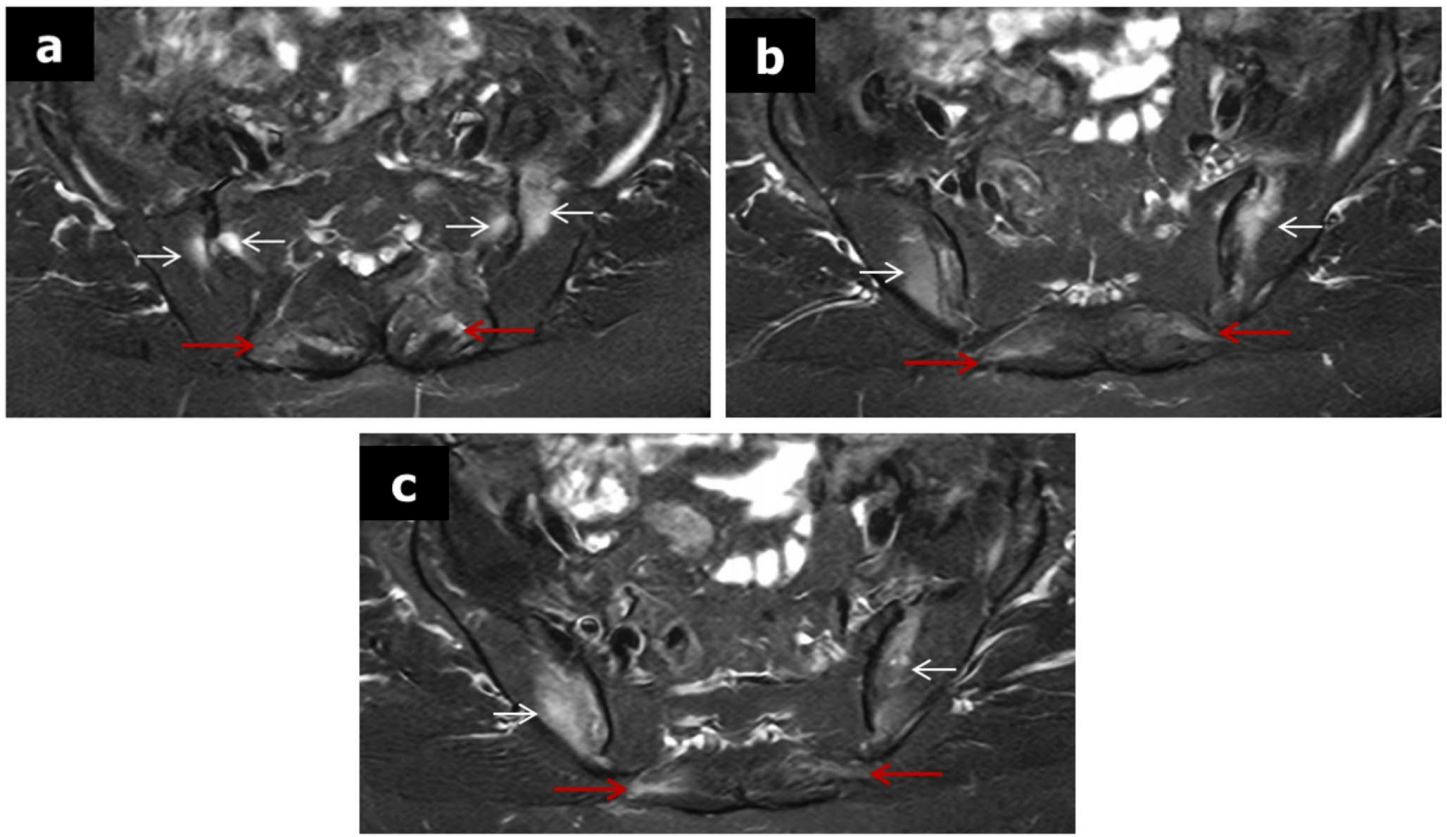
Sacroiliac joints axial MR images (a, b,c) T2-weighted fat suppressed images show bilateral subchondral bone marrow edema (white arrows) and enthesitis (red arrows).
